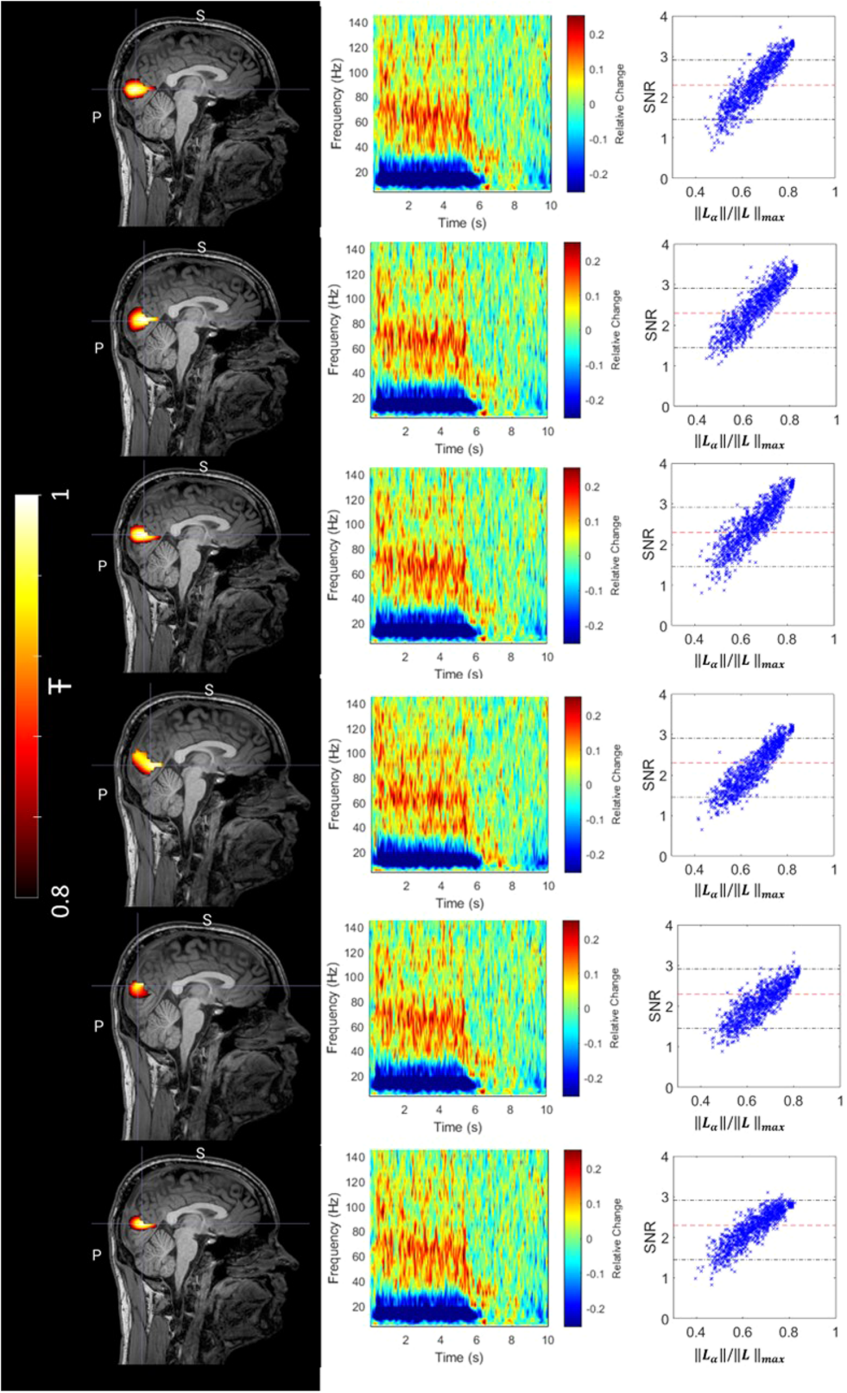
Results from the naturalistic viewing task. The separate rows show six independent experimental repeats. The left-hand panel shows the pseudo-T-statistical image depicting maximum regions of gamma modulation, overlaid onto a subject specific anatomical MRI. The functional image was thresholded at 80% of its maximum. The centre panels show a TFS, extracted from the average peak location (across runs). The right-hand panels show SNR plotted as a function of$\left\|{\text{L}}_{\alpha }\right\|$. Here,$\left\|{\text{L}}_{\alpha }\right\|$was altered by randomly removing different channels. Blue dots represent each iteration of random sensor array designs, and the red dashed and black dotted-dashed lines show the mean and range of the SNR values obtained using cryogenic MEG respectively. An equivalent figure for the grating experiment is shown inSupplementary Figure S2.
